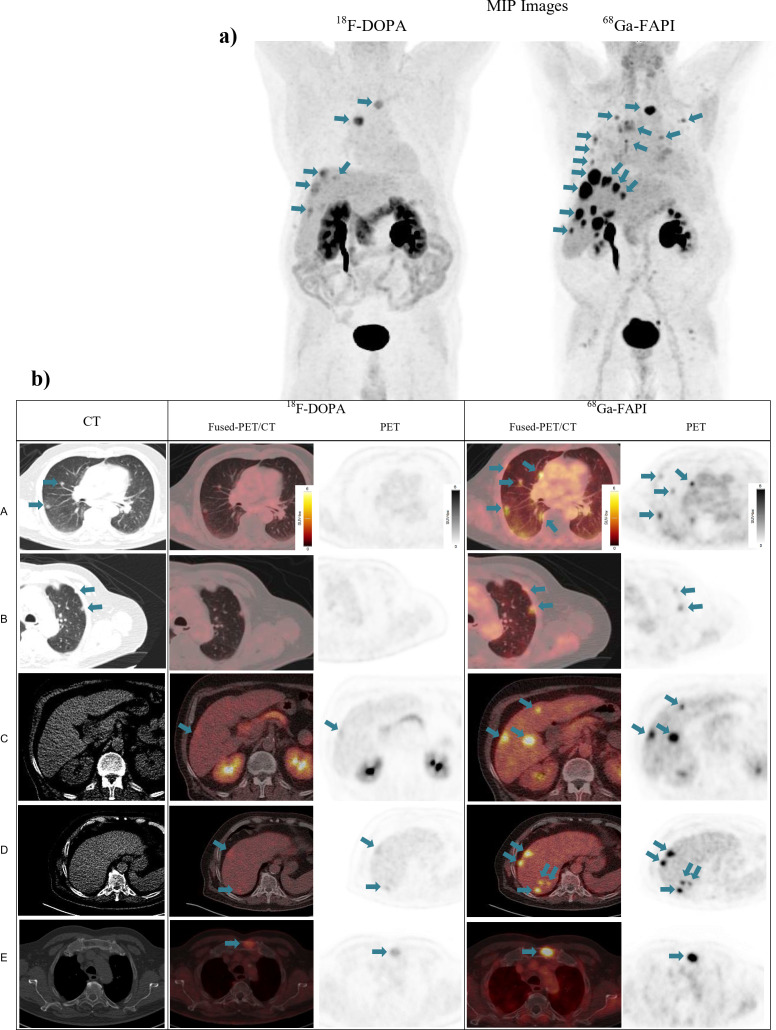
A 68-year-old male patient with sporadic MTC (pT1b) presented with an elevated calcitonin level (158 pg/ml) and subsequently underwent ^18^F-DOPA and ^68^Ga-FAPI PET/CT. This figure displays the resulting images: (A) maximum intensity projection (MIP), (B) CT, (C) fused PET/CT, and (d) PET. Rows (A, B) reveal multiple lung metastases (arrows) exhibiting strong ^68^Ga-FAPI uptake (SUVmax = 8.4, TBR = 11.3) in contrast to the absence of ^18^F-DOPA uptake. Similarly, Rows (C, D) demonstrate multiple liver metastases (arrows) with strong ^68^Ga-FAPI uptake (SUVmax = 9.9; TBR = 3.4) where no ^18^F-DOPA uptake was observed. Row (E) shows a bone metastasis in the manubrium of the sternum (arrows).
